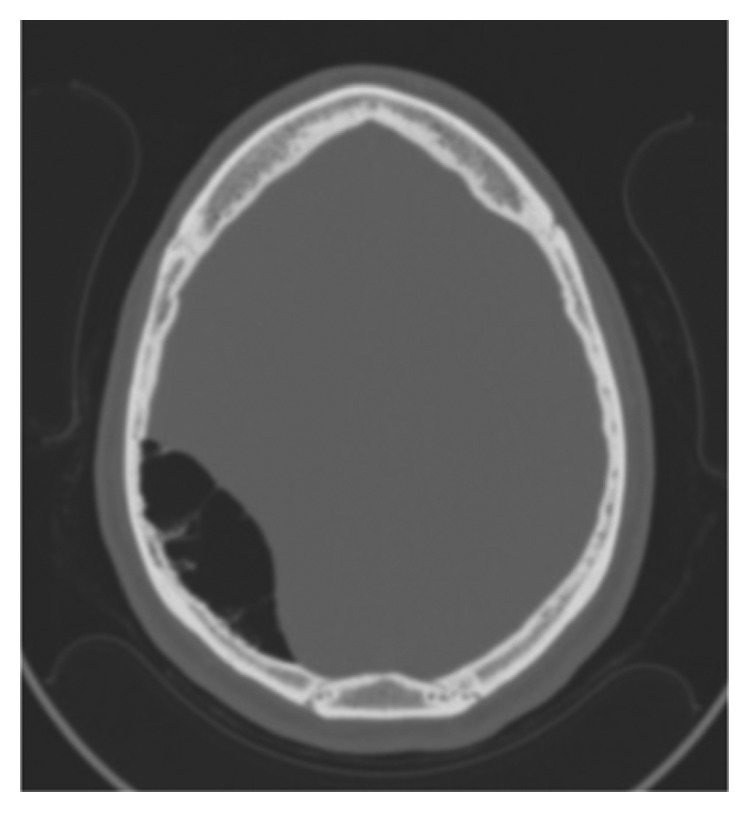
CT head in bone window demonstrating pneumocephalus.
